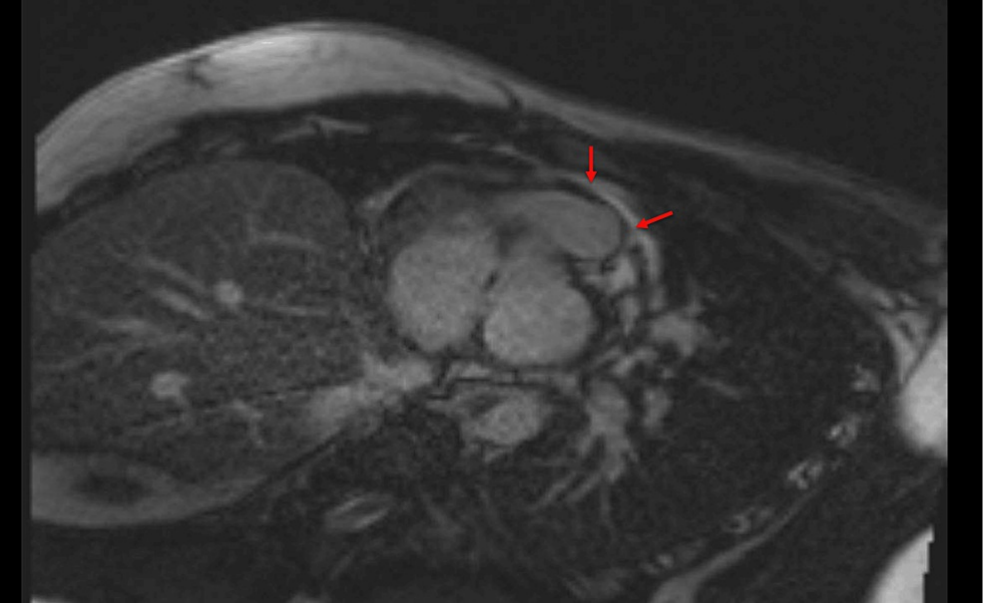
Cardiac Magnetic Resonance imaging showing mid-wall myocardial enhancement at the basal anteroseptal wall and basal anterolateral wall (see red arrows)
